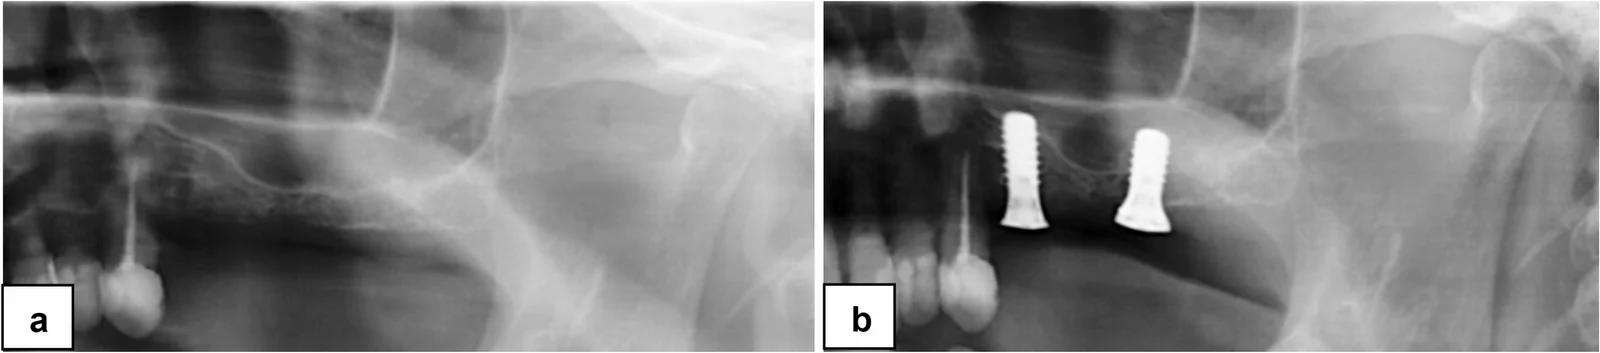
Panoramic x-ray of implantation in the upper leftsided jaw, (a) bone height before implantation, (b) after implantation (regio 024: one-stage internal elevation, regio 026: one-stage external elevation)
